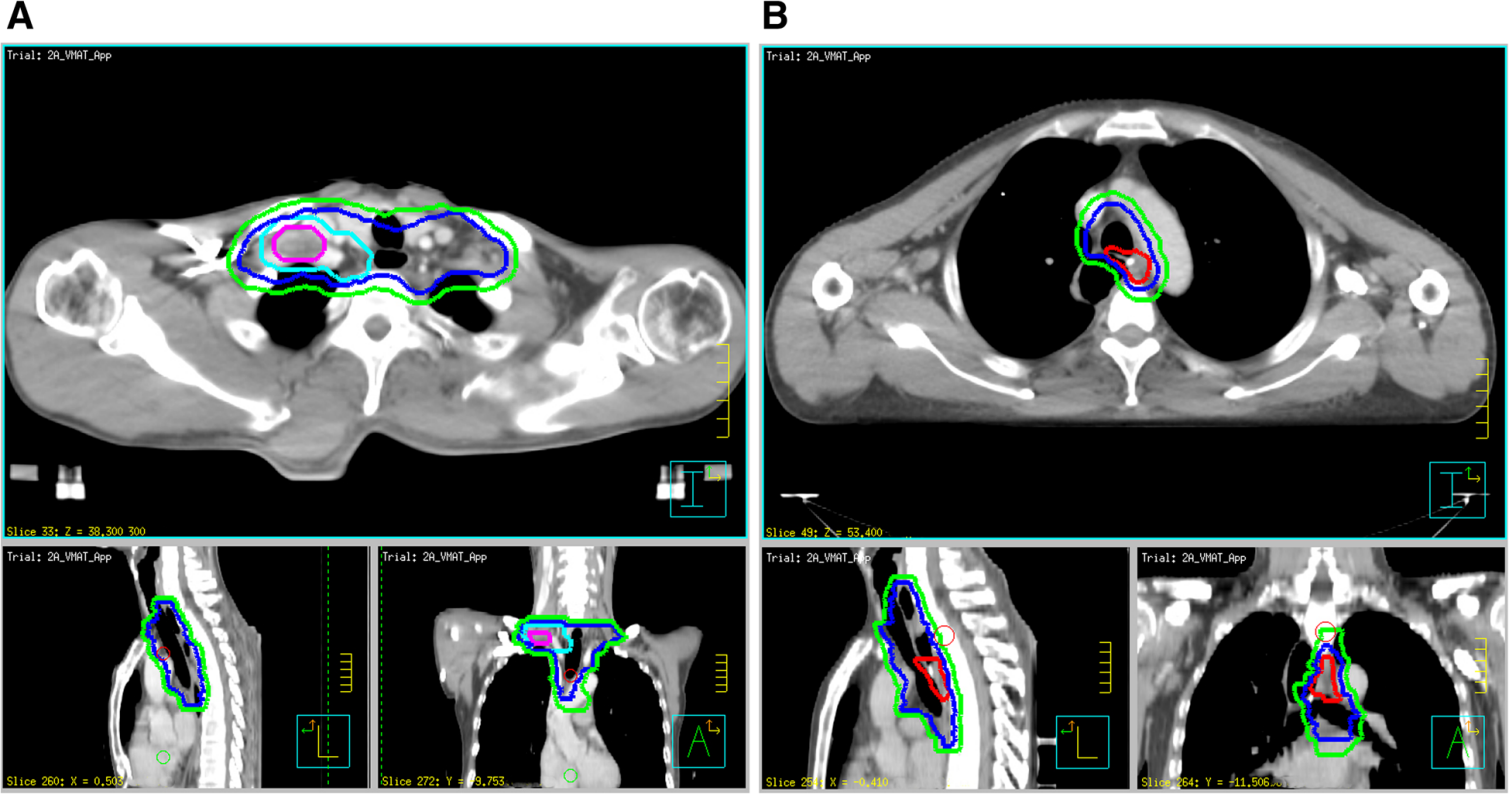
Examples of target volumes delineated in elective nodal irradiation (ENI) pattern are shown based on the planning computed tomography (CT) scans. a Lesions far from the thoracic stomach recurred in right supraclavicular space. The ENI field includes bilateral supraclavicular space, 1R/L, 2R/L, 4R/L, partial 7, and 8 U/M. b Lesions adjacent to the thoracic stomach recurred in Station 8 U, ENI field includes bilateral supraclavicular space, 1R/L, 2R/L, 4R/L, partial 7, and 8 U/M. Pink outlines GTV-N, Red outlines GTV-T, blue outlines CTV, sky blue outlines PGTV, and green outlines PTV
